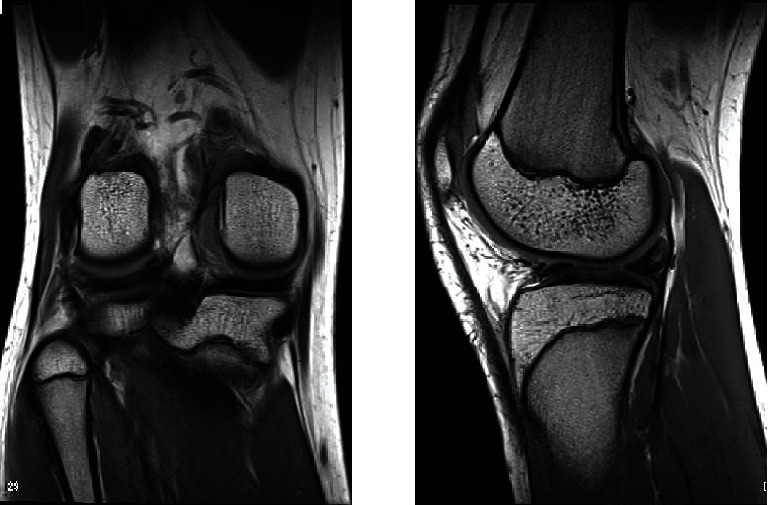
Magnetic resonance imaging (proton density-weighted image) shows high signal in the posterior segment and an increase in its volume.
